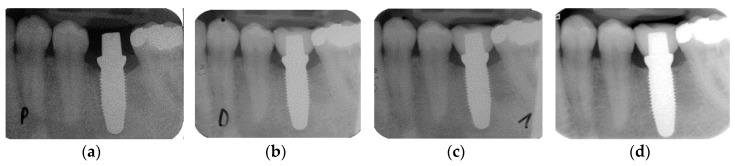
Standardized radiographs for the assessment of marginal bone loss (MBL): (a) implant insertion; (b) prosthetic delivery; (c) one-year follow-up; (d) five-year follow-up.
